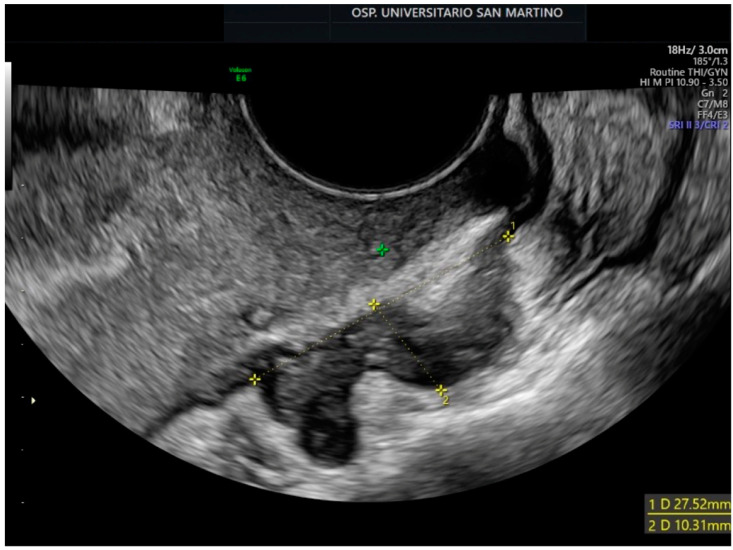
The figure displays the presence of an endometriosis implant on the rectum in a patient undergoing a transvaginal ultrasound at our San Martino Hospital in Genoa. This patient experienced symptoms including dyschezia, rectal tenesmus, alternating episodes of constipation and diarrhea, as well as painful intestinal spasms. The yellow calipers represent the measurements. The green one represents the ultrasound machine’s pointer.
